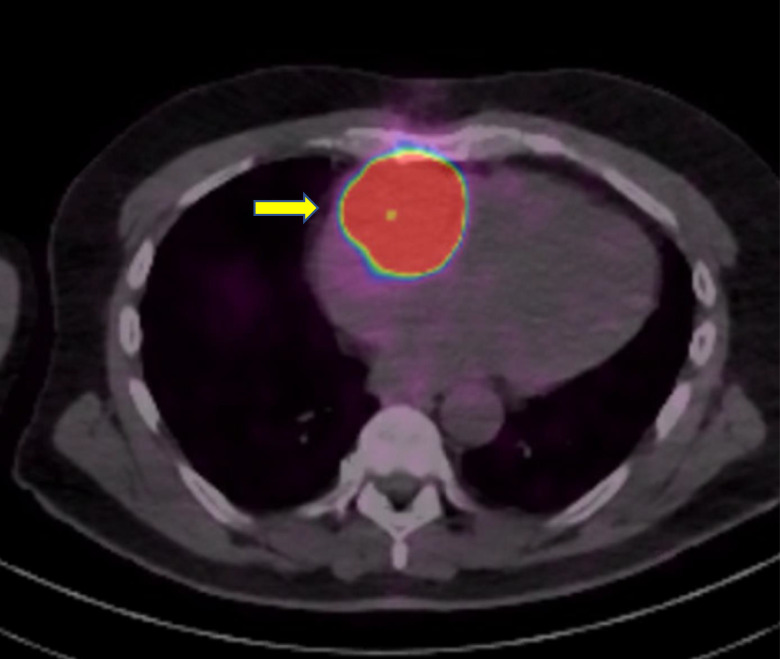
Initial DOTATATE PET/CT: DOTATATE avidity within the region of the right-sided cardiac mass. SUVmax 94.
